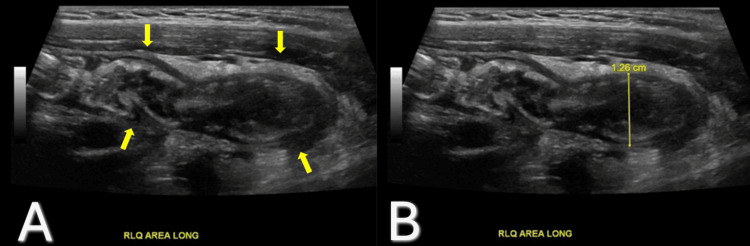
(A) Ultrasound imaging with arrows pointing to a dilated appendix with a (B) maximum diameter of 12.6 mm.
